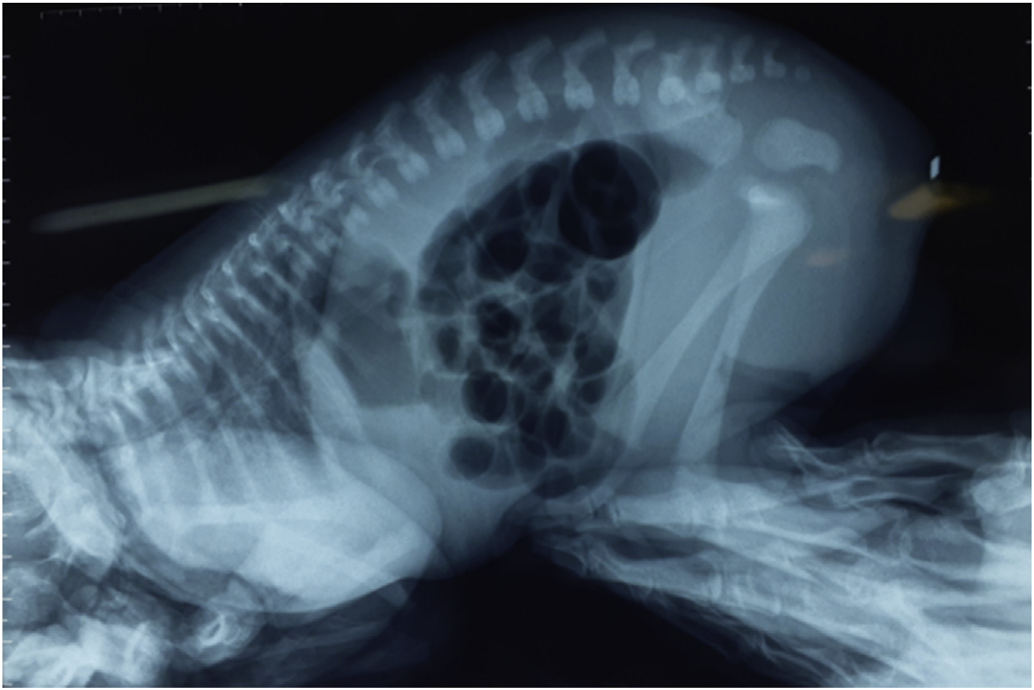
A plain cross-table lateral x-ray film with the newborn in prone position.
